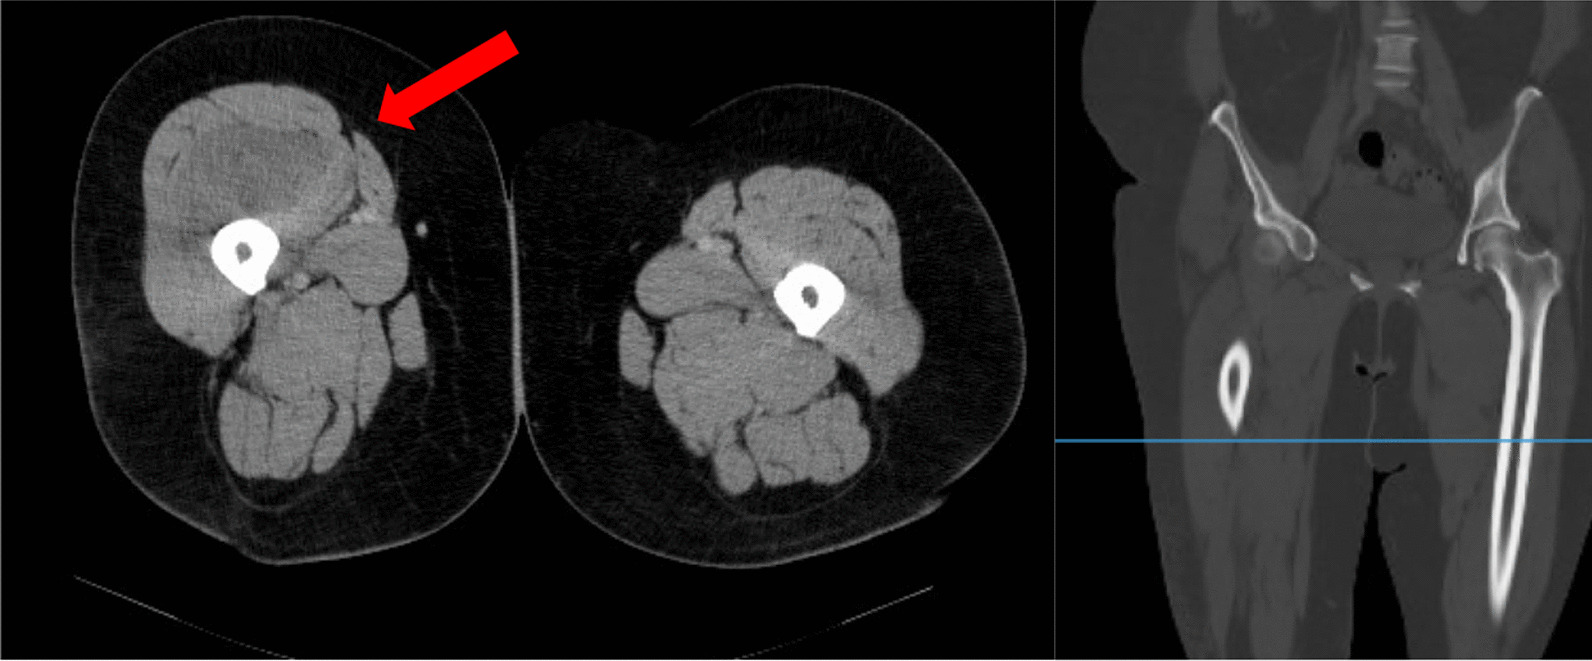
CT pelvic and leg imaging with contrast medium from postoperative day 2 (red arrow: clear signal alteration and circumferential increase in the vastus intermedius muscle in a side-by-side comparison)
